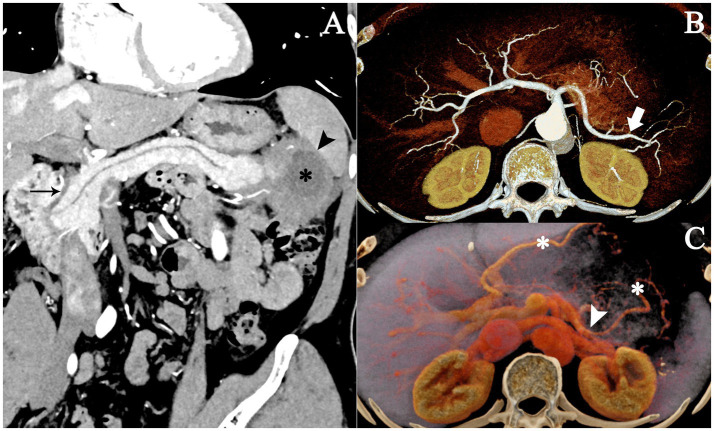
Curved planar and volume-rendered reconstructions from contrast-enhanced CT. (A) Curved planar reconstruction (PAP, 1 mm slices) reveals a cystic-solid lesion in the pancreatic tail (black asterisk) with an irregular contour abutting the spleen (black arrowhead) and obscuration of the fat plane. The common bile duct appears slender and smooth (thin black arrow), without typical features of IgG4-related disease. (B,C) Cine volume-rendered reconstructions (0.4 mm PAP and PVP images) clearly delineate the celiac trunk and major arterial branches, as well as the portal venous system. The lesion encases the distal splenic artery (solid white arrow) and splenic vein (white arrowhead). The distal splenic artery and its branches exhibit mild luminal narrowing, while the splenic vein gradually tapers toward the hilum to complete occlusion, accompanied by multiple collateral veins (white asterisks). PAP, pancreatic parenchymal phase; PVP, portal venous phase.
